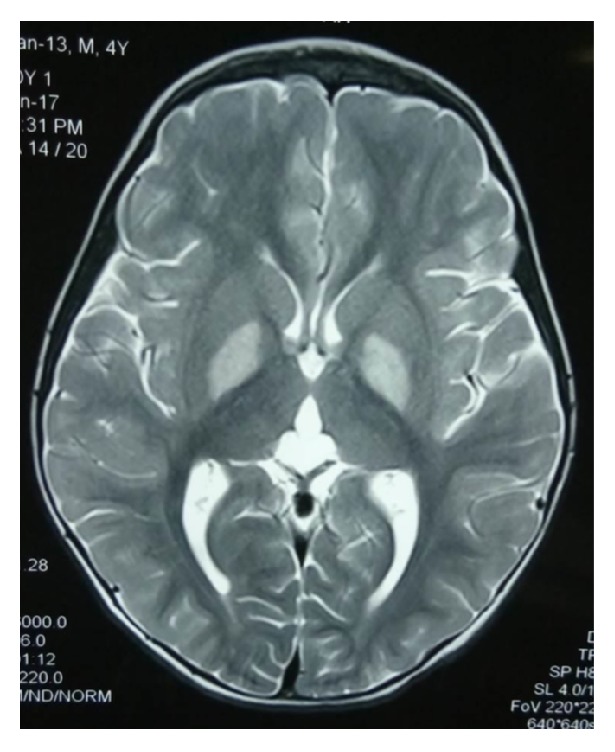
The MRI brain in the child with MUT-related MMA showing predominant frontoparietal abnormalities in form of encephalomalacia and gliosis.
